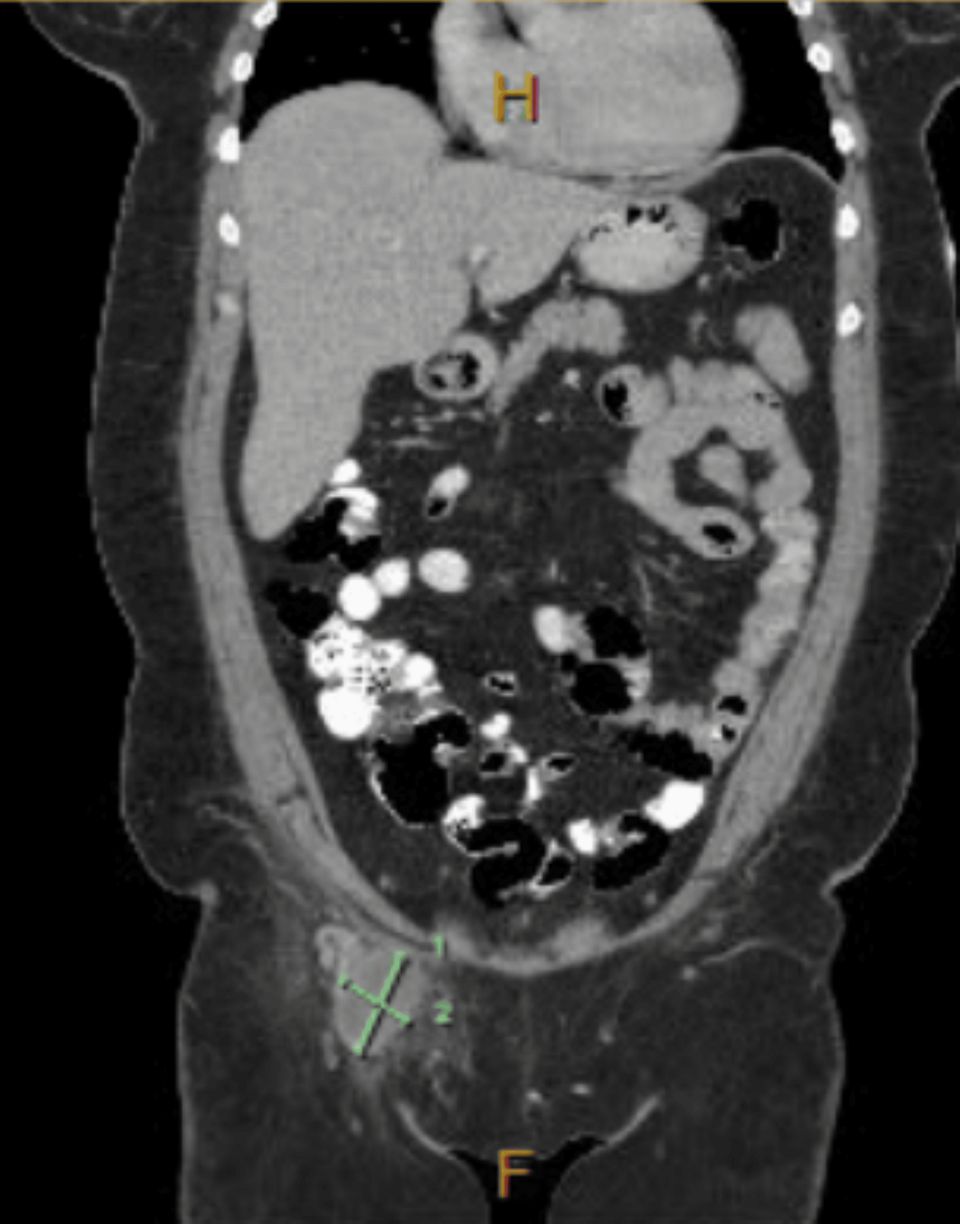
Coronal section of abdomen MRI, the green markers showing the large inguinal lymphadenopathy that measured 3.5 cm x 4.9 cm.
